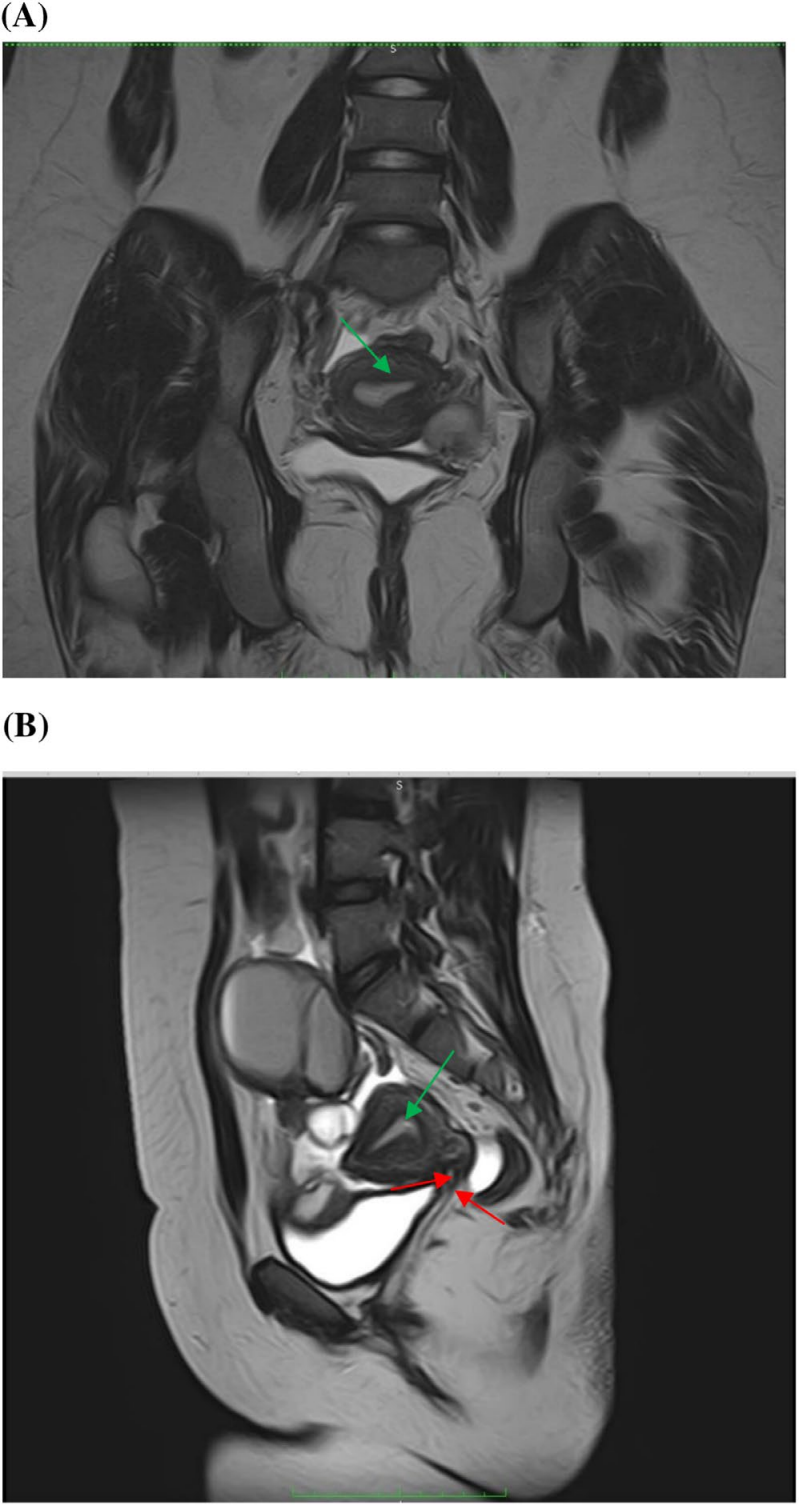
(A) Coronal T2 (B) Sagittal T2. MR imaging findings showing cervical hypoplasia and vaginal agenesis (red arrows) and expanded uterine cavity (green arrows).
